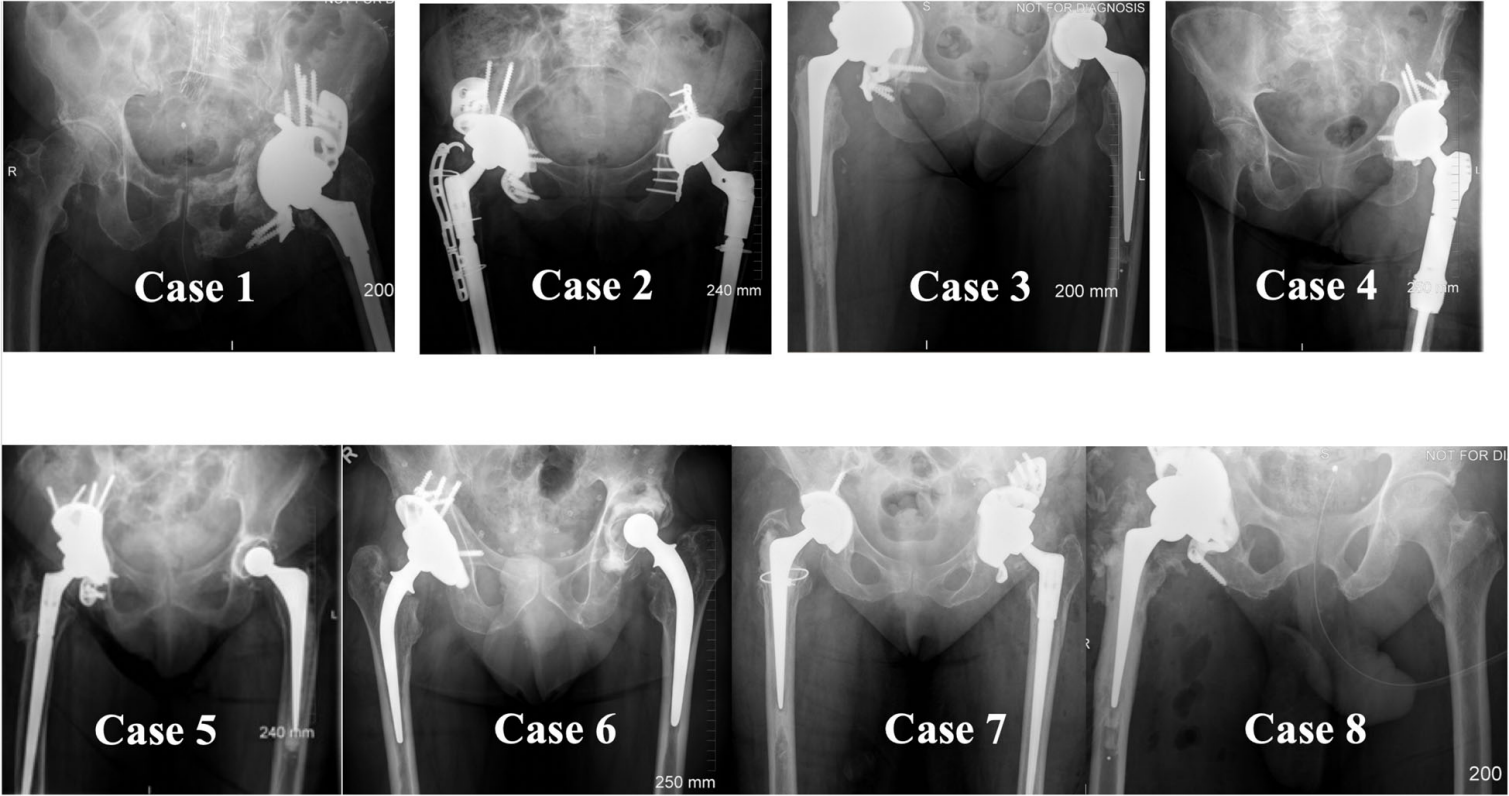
Post-operative radiographs of the 8 patients with the custom metal work in place
